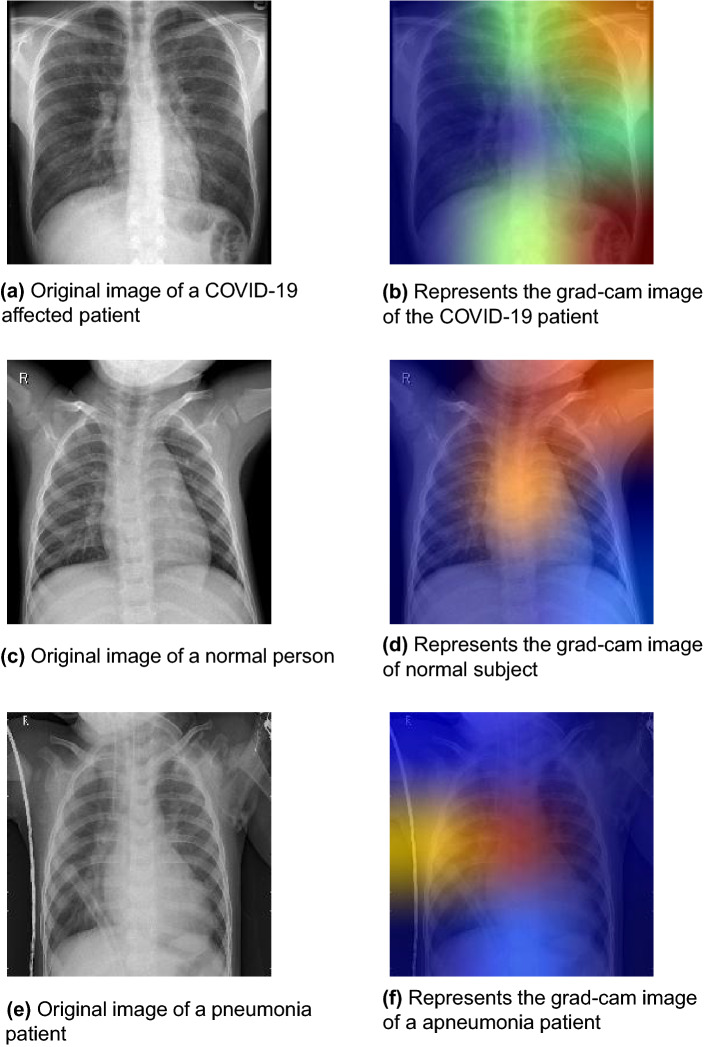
Grad-cam images along with original chest X-ray images for the base classifier 2 trained on the Dataset-1. Images are taken from the public datasets found in^51,52^.
